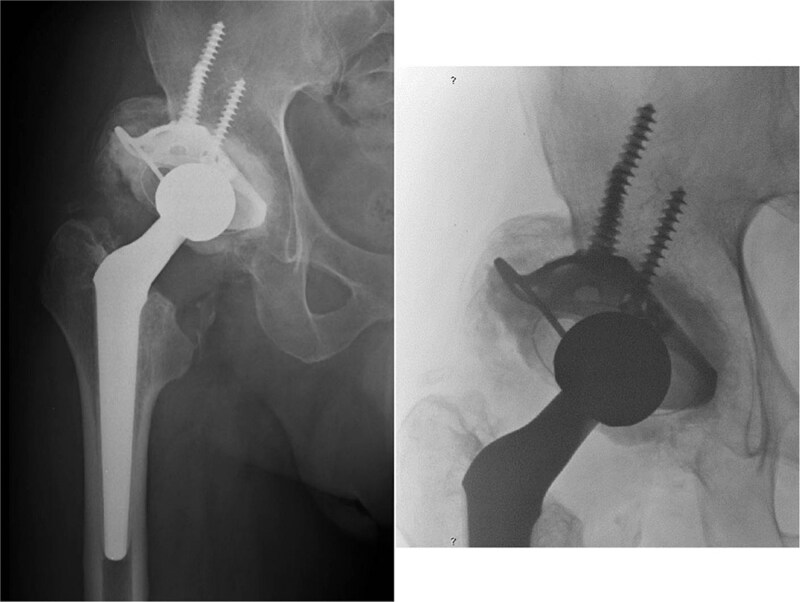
Postoperative THA radiograph at 1-year follow-up. A significant superolateral portion of the roof reinforcement ring remains uncovered by the native host bone. Evidence of the remodelling process is visible in the impacted morselised bone autograft, situated between the metal ring rim and the superior margin of the dysplastic acetabulum.
